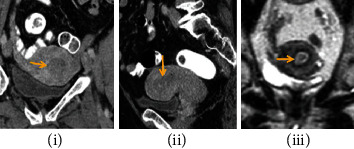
Multilayer spiral CT analysis.
